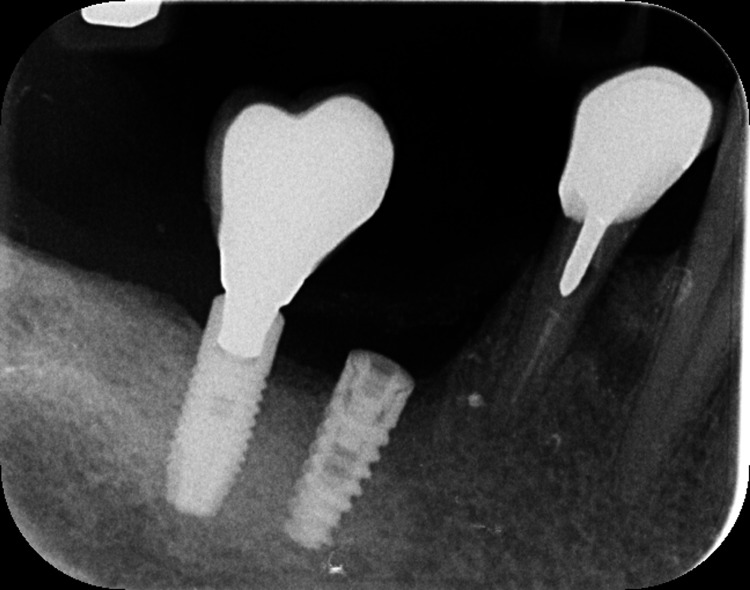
Preoperative radiograph showing LPC adjacent to tooth #44 LPC, lateral periodontal cyst
